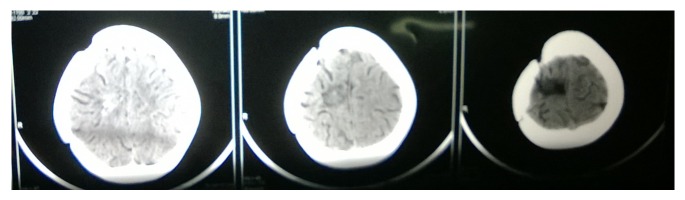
CT scan brain with residual tumor in the right temporoparietal region May 2014.
